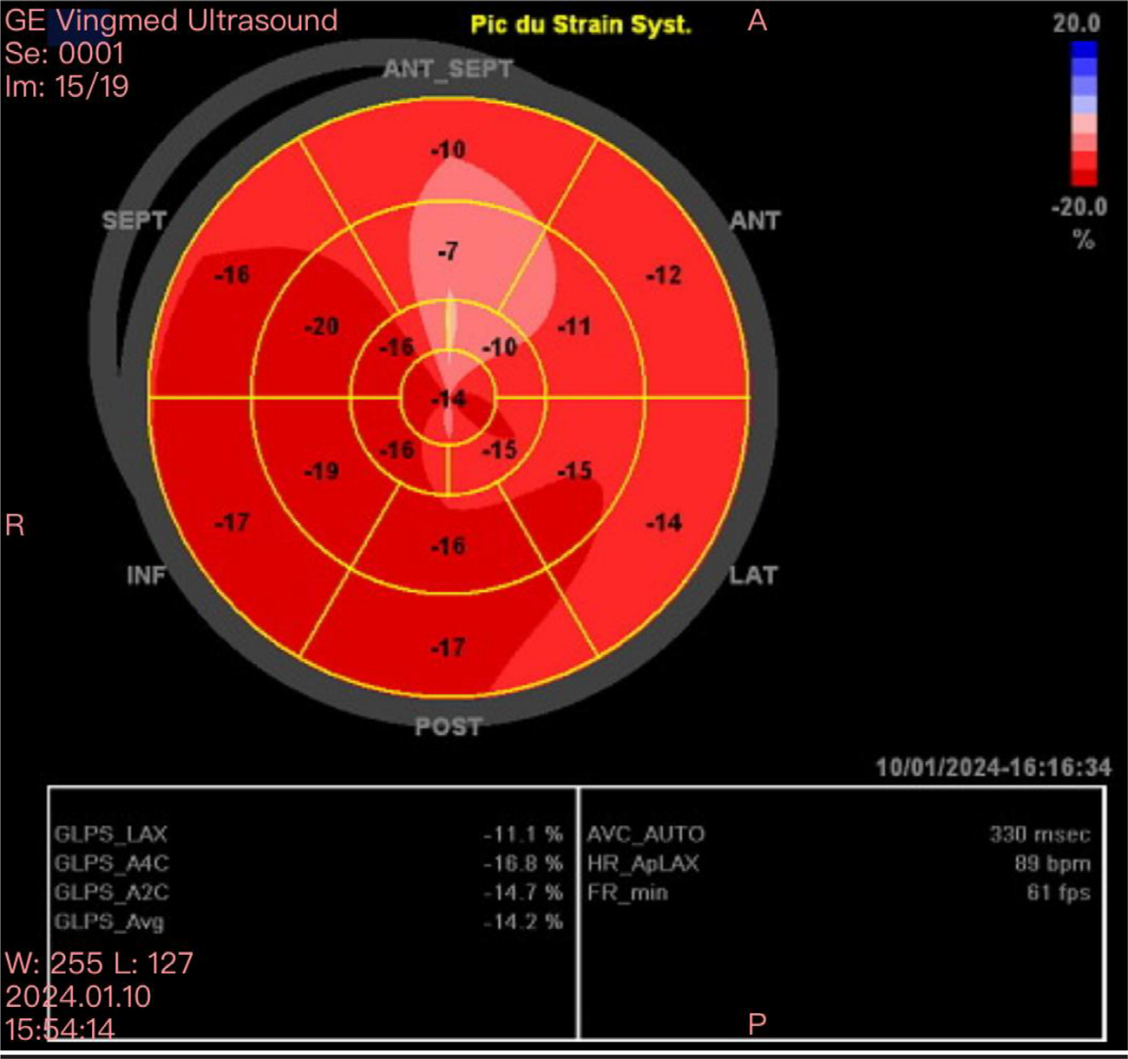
Echocardiography (ETT) showing global longitudinal strain reduced to −14%, at the expense of lateral and anterior walls.
